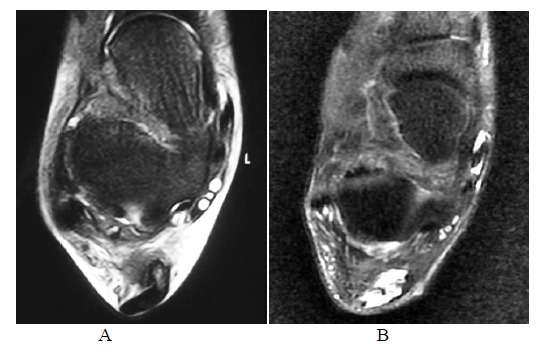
Pre-operative and 2-month post-operative MRI in Patient C with partial Achilles tear. Almost the entire cross sectional area of the Achilles tendon appears T2-weighted hyperintense, even in its previously intact portion.
